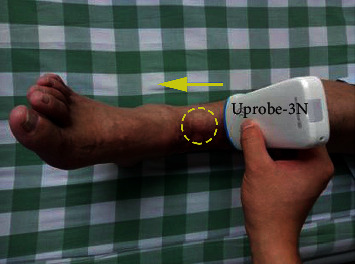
Ultrasound scanning of the right distal tibia using Uprobe-3N, a wireless ultrasound probe. The arrow indicates the scanning orientation. The dotted ellipse presents the site of LIPUS therapy.
